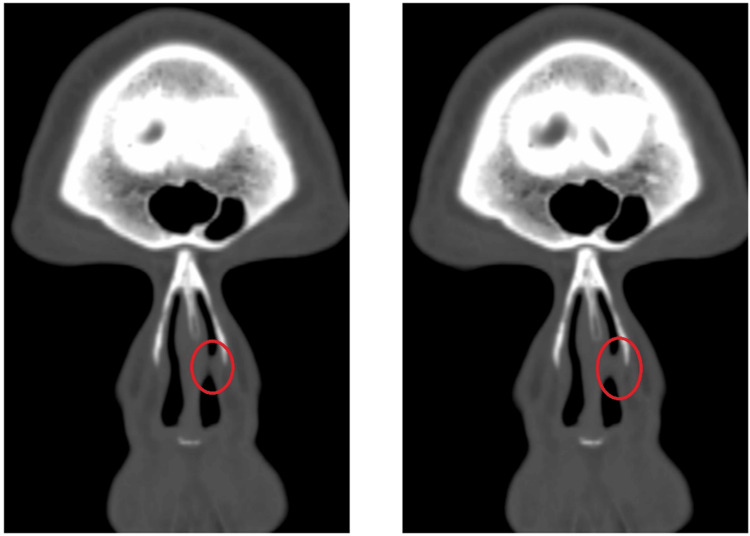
Synechia after surgery in the nasal cavity of patient 4 on two CT slices. Author's own creation
